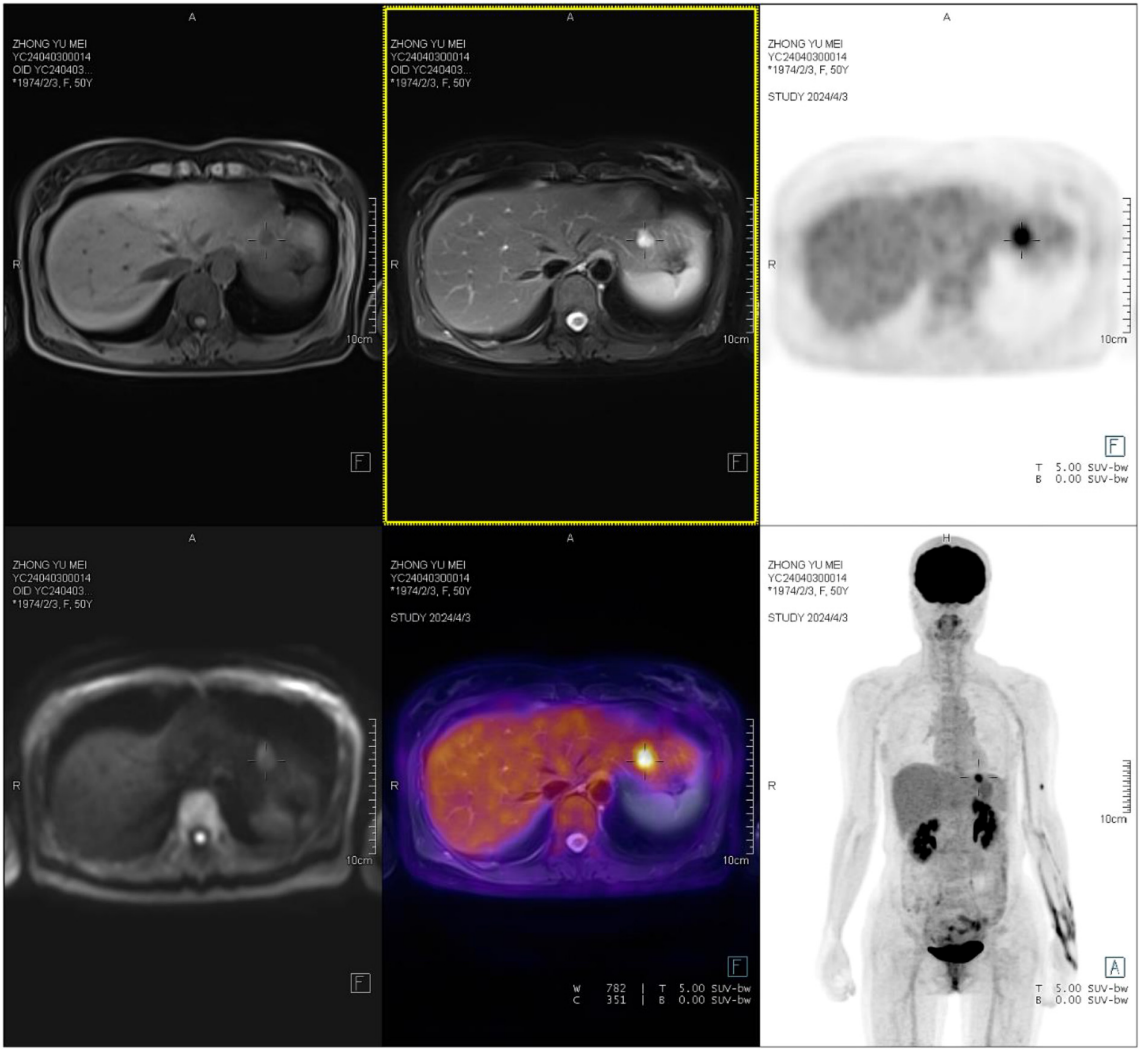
A low-density nodule is observed in liver segment II, with slightly low T1WI signals and slightly high T2WI signals. The nodule has clear boundaries and measures approximately 1.2×1.1 cm, with increased FDG uptake and an SUVmax of 8.4.
